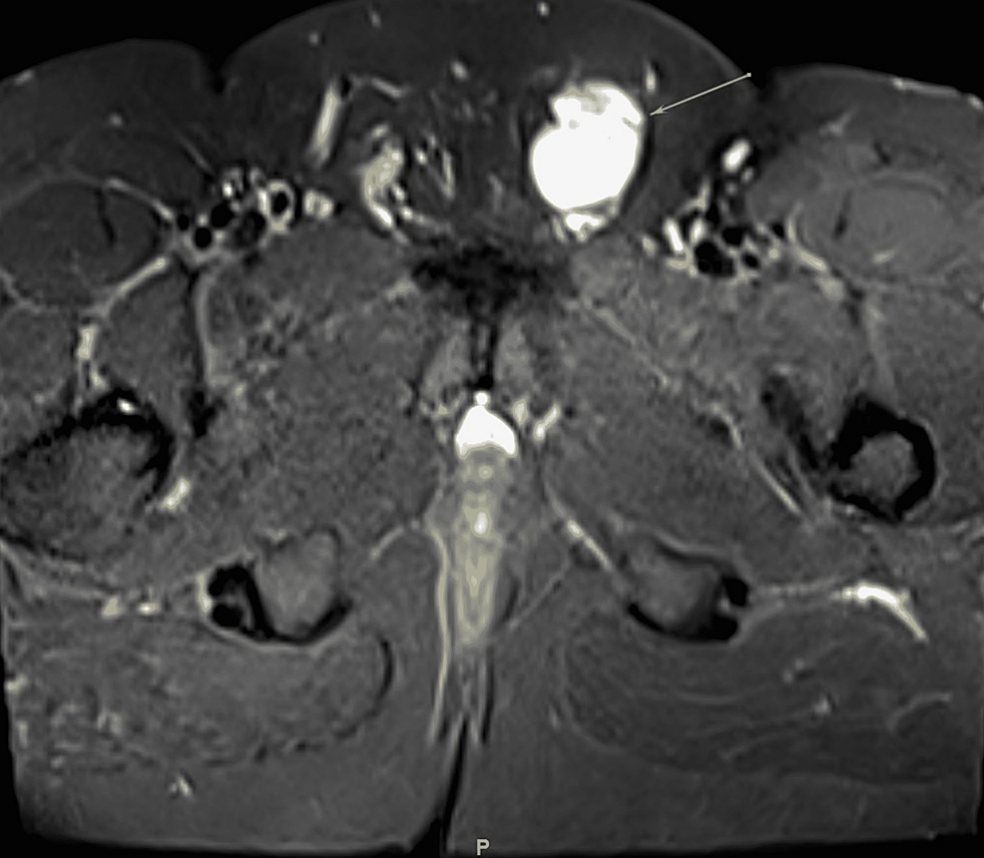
Cross-section T2-weighted MRI. No internal fatty or fibrous component. There is an intermediate to high signal intensity with intense homogeneous enhancement postcontrast administration. Unremarkable appearance of bilateral testicles and epididymis. No enlarged inguinal lymph nodes. Unremarkable urinary bladder, seminal vesicles, and prostate gland.
